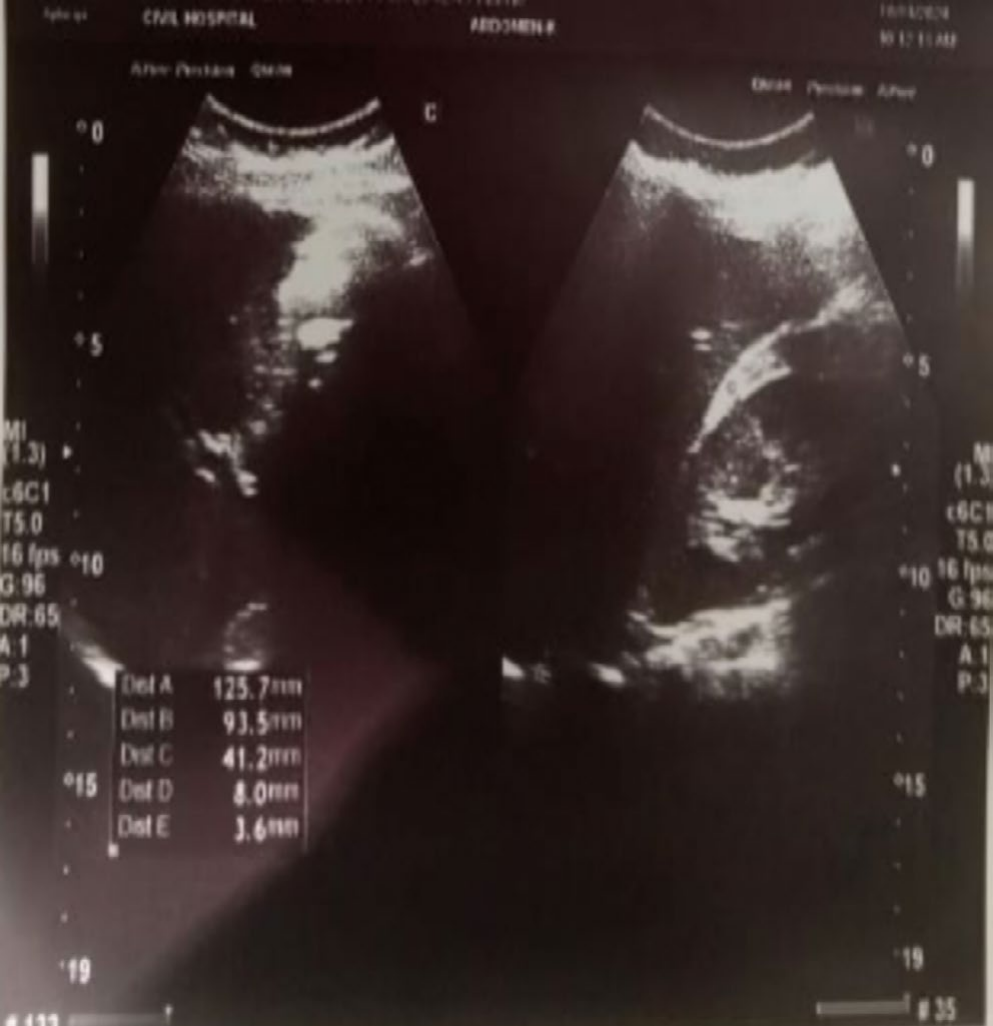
Abdominal ultrasound suggested no dilatation of duct with normal diameter of common bile duct (CBD) of 0.3 cm.
